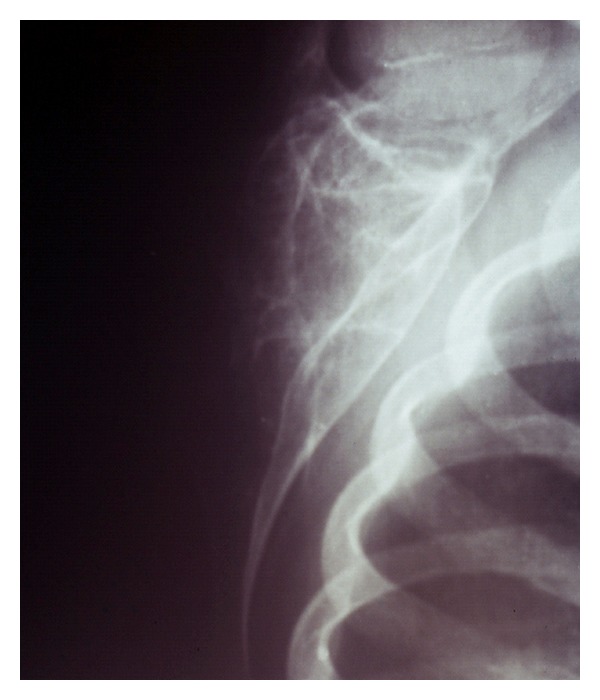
Postoperative scapular view radiograph of the right scapula. Five-year followup.
